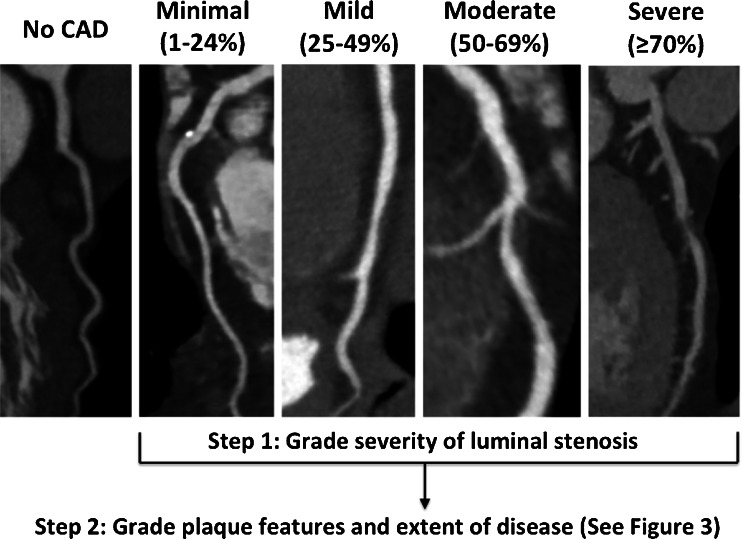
Coronary CTA identified CAD severity. Recommended quantitative stenosis grading of CAD assessed by coronary CTA [40]. Following stenosis assessment, recommend grading of CTA-identified plaque features and CAD extent (see Fig. 3). *CAD*, coronary artery disease; *CTA*, computed tomographic angiography
